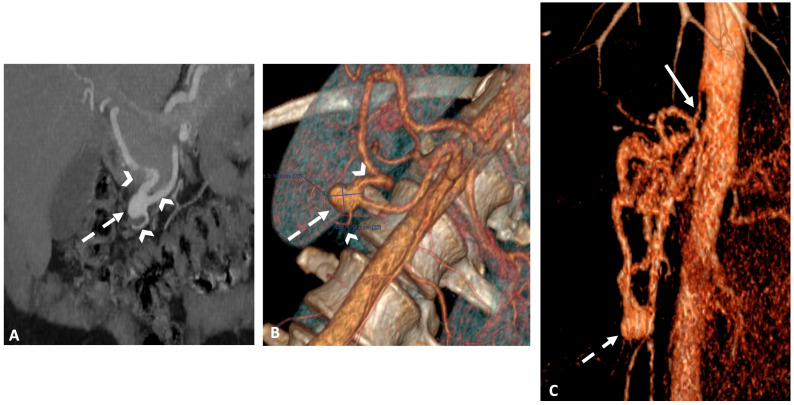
Abdominal CT after contrast injection at the arterial phase in a coronal view with maximum intensity projection reformatting (A), oblique 3D volume rendering (B), and sagittal 3D volume rendering (C) reconstructions showing a tight stenosis at the origin of the celiac trunk due to compression by the median arcuate ligament (solid arrow, C) and an unruptured aneurysm of the pancreaticoduodenal arcade (dashed arrow, (A–C)), as well as aneurysm’s inflow and outflow tracts (arrowhead, (A,B)).
